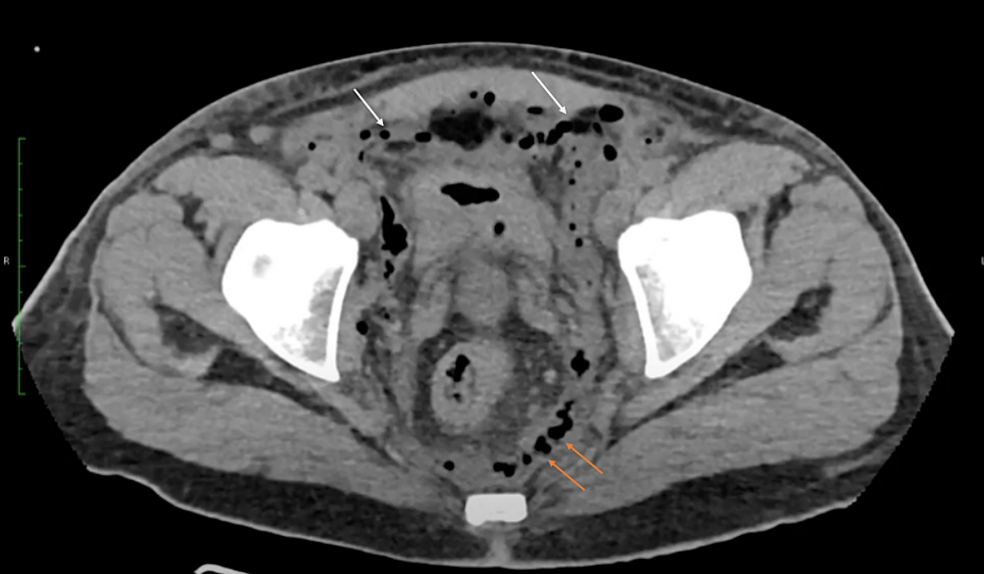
Non-contrast abdominal CT in axial view. Intraperitoneal emphysema (white arrows) with retroperitoneal extension (orange arrows). These findings are consistent with the intraperitoneal and retroperitoneal extension of FG. CT: computed tomography; FG: Fournier’s gangrene
